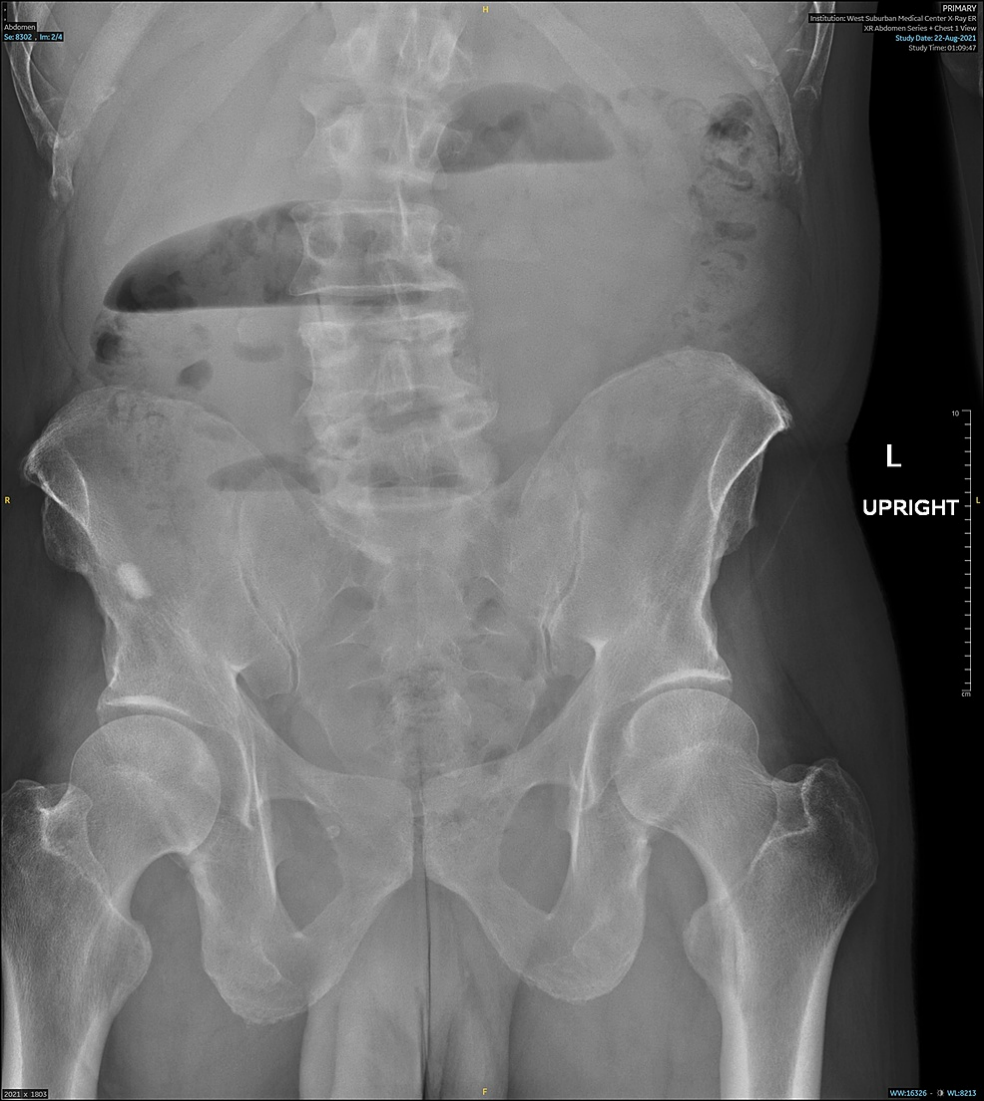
Abdominal radiograph showing adynamic air-fluid levels.
